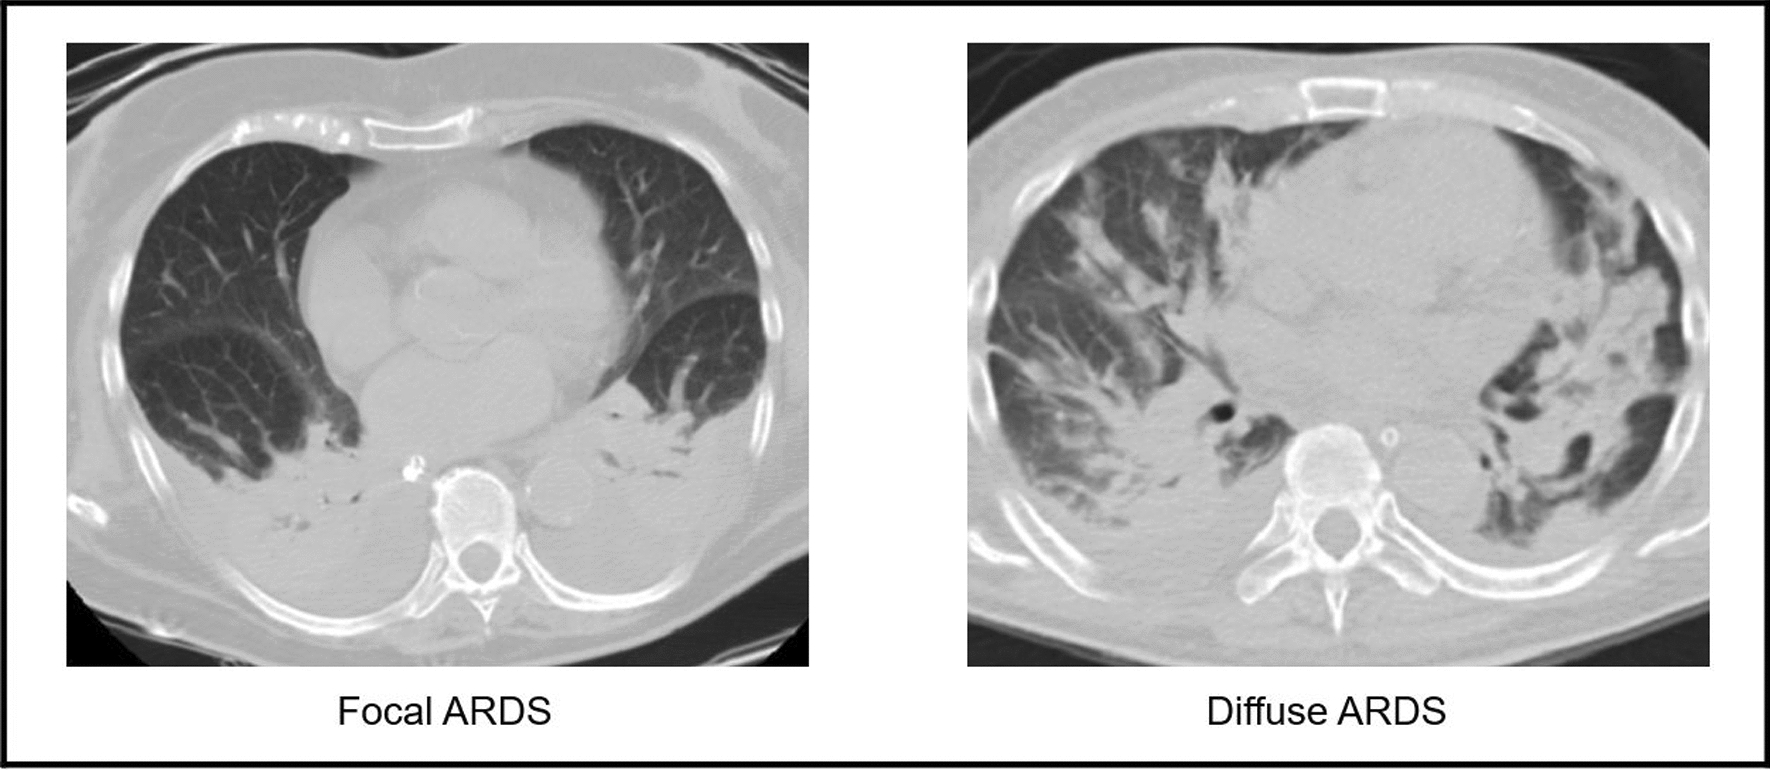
Two CT scans showing ARDS focal pattern on right panel and diffuse pattern on left panel
